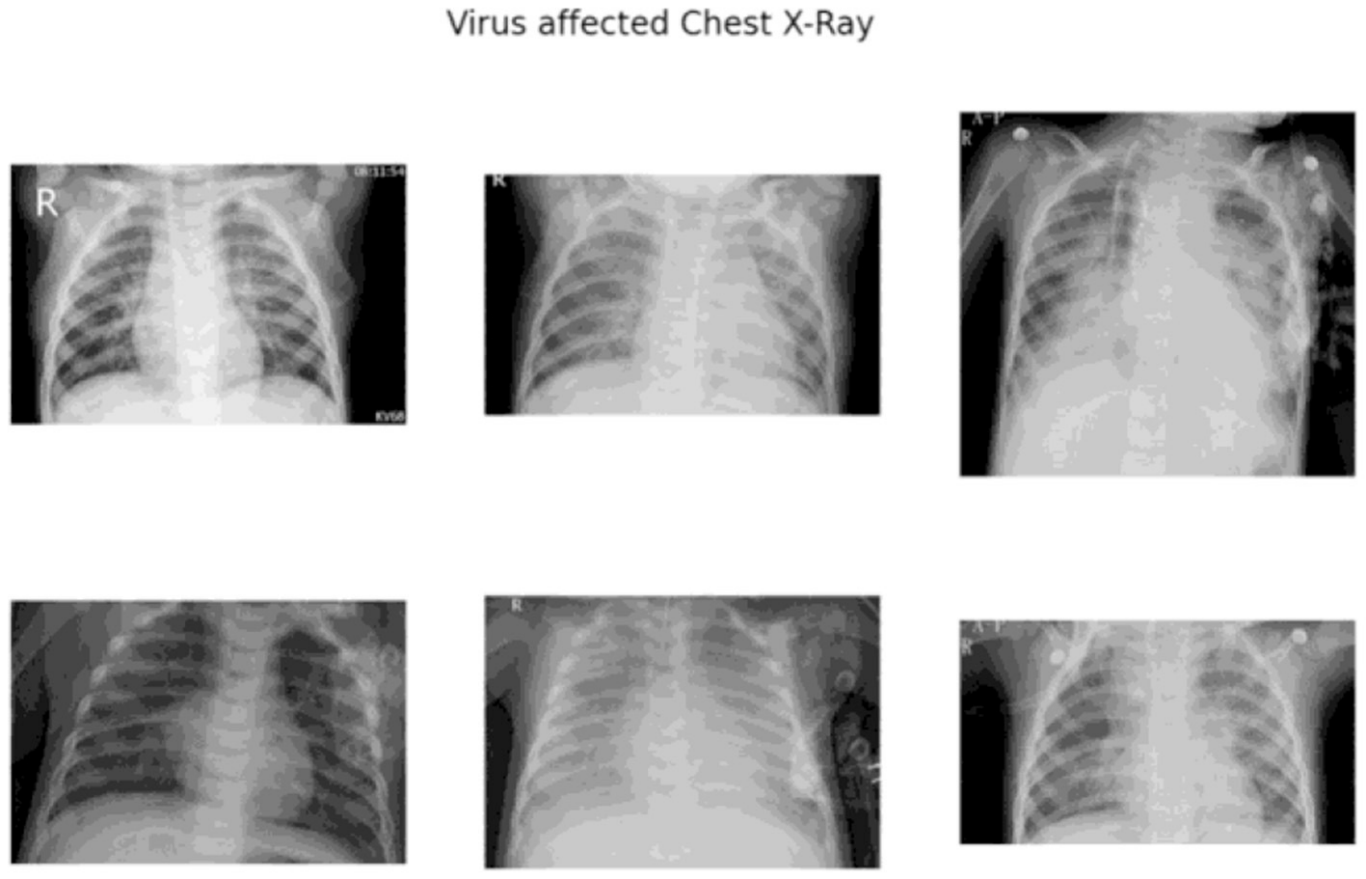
Chest X-ray Pneumonia image samples evaluated using the Kaggle database.
